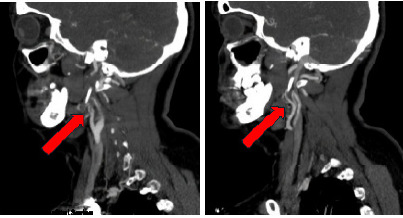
Computed tomography angiography of the neck in sagittal view showing bilateral internal carotid artery dissection (red arrows).
